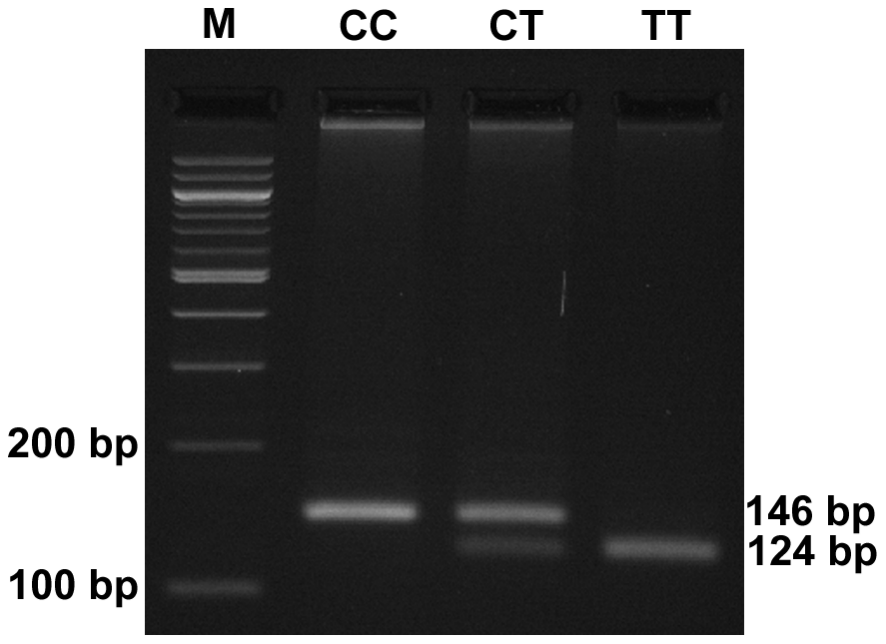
Genotyping of *MTHFR* C677T by PCR-RFLP. Substitution of cytosine (C) by thymine (T) at *MTHFR* C677T creates a restriction site for *HinfI* (5′G/ANTC3′), therefore the PCR product with CC genotype remains undigested and appears as a 146 bp band on a 4% agarose gel. The CT genotype allows the 146 bp amplicon to be digested into two fragments of 124 bp and 22 bp, and hence produces three fragments of 146 bp, 124 bp and 22 bp. However, the 4% gel cannot resolve the 22 bp fragment and only the two higher bands are visible. A single band of 124 bp is inferred as TT genotype [28]. M: 100 bp DNA Ladder (NEB).
